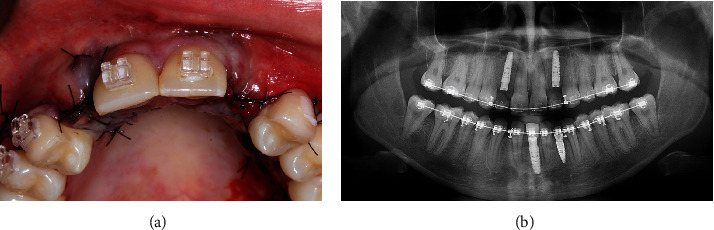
(a) Immediate postoperative view. (b) Radiographic examination showing the parallelism and osseointegration of the dental implants and bone healing.
